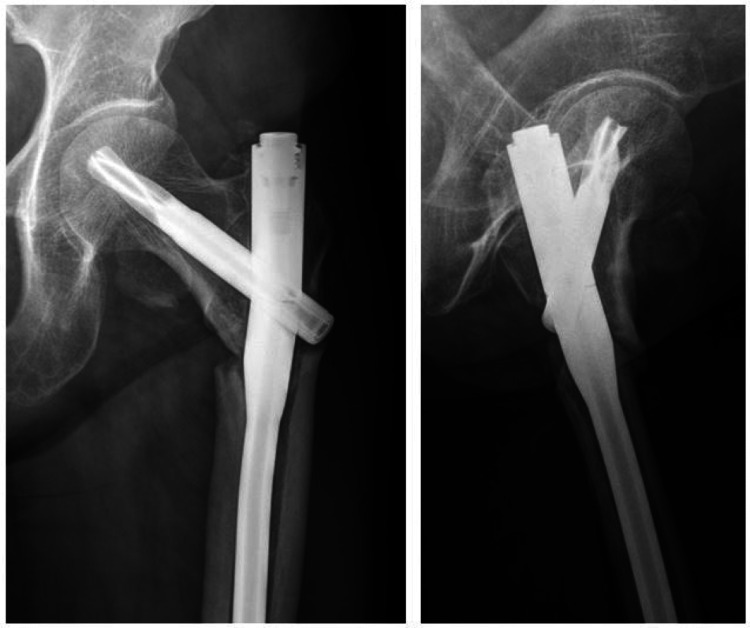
Anteroposterior and lateral radiographs of the left femur obtained 15 months after index surgery shows nail breakage which indicates nonunion.
